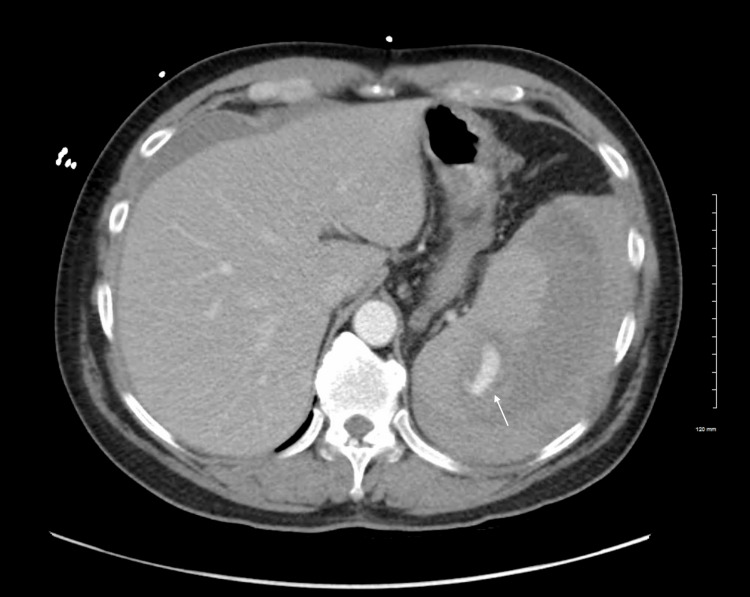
Axial CT of the abdomen and pelvis with contrast demonstrating a focal area of acute hemorrhage in the posterior aspect of the spleen measuring 2.8 × 1.4 cm
